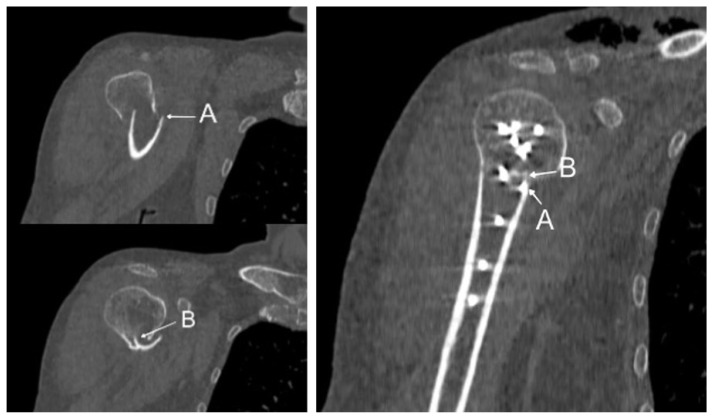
Pre- and postoperative measurement of the medial hinge on computed tomography (measured as the distance between the medial cortex in the section where the most proximal end of the distal part (diaphysis) of the fracture is seen widest in the coronal plane and the medial cortex of the most distal end of the proximal part in the section where the proximal part (humeral head) is seen most spherically in the coronal plane).
